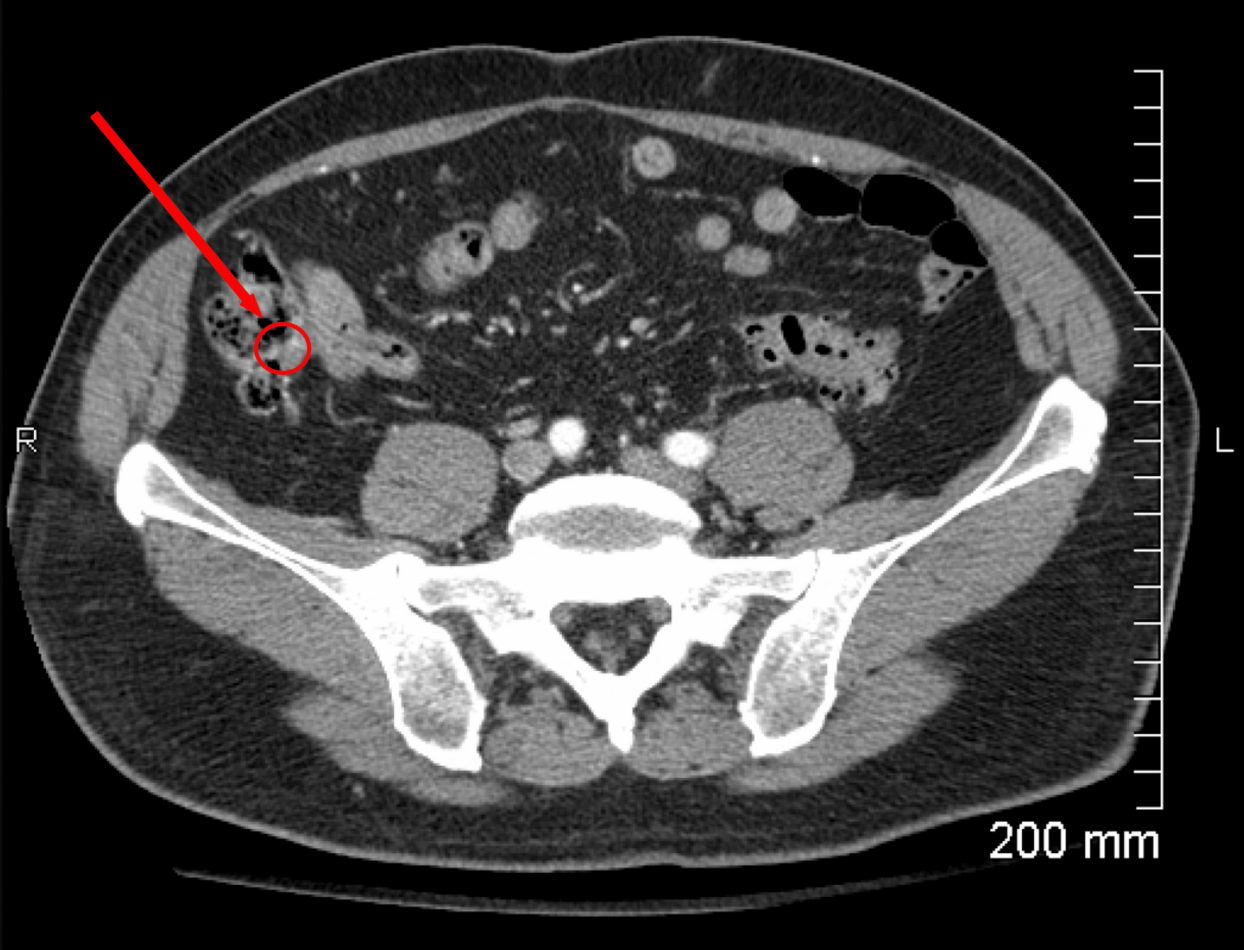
Contrast-enhanced CT of the abdomen and pelvis Axial CT demonstrates a mildly dilated appendix measuring 13 mm with periappendiceal fat stranding. The area of interest is denoted by the red arrow, consistent with chronic appendiceal inflammation CT, computed tomography
